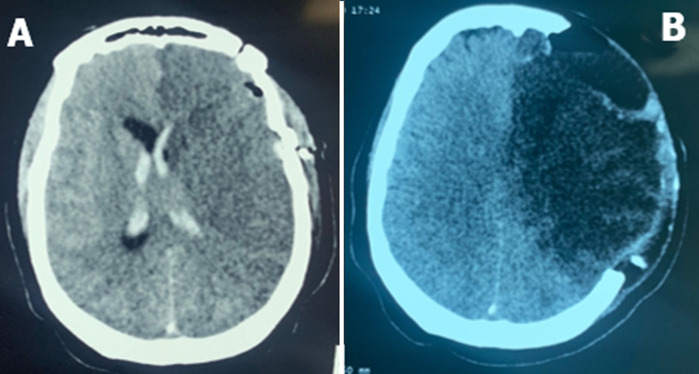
A) axial section of a brain CT scan of a post-exercise ischemia of an olfactory meningioma; B) cerebral CT scan axial section post-craniectomy decompressive ischemia post-exeresis of olfactory meningioma
